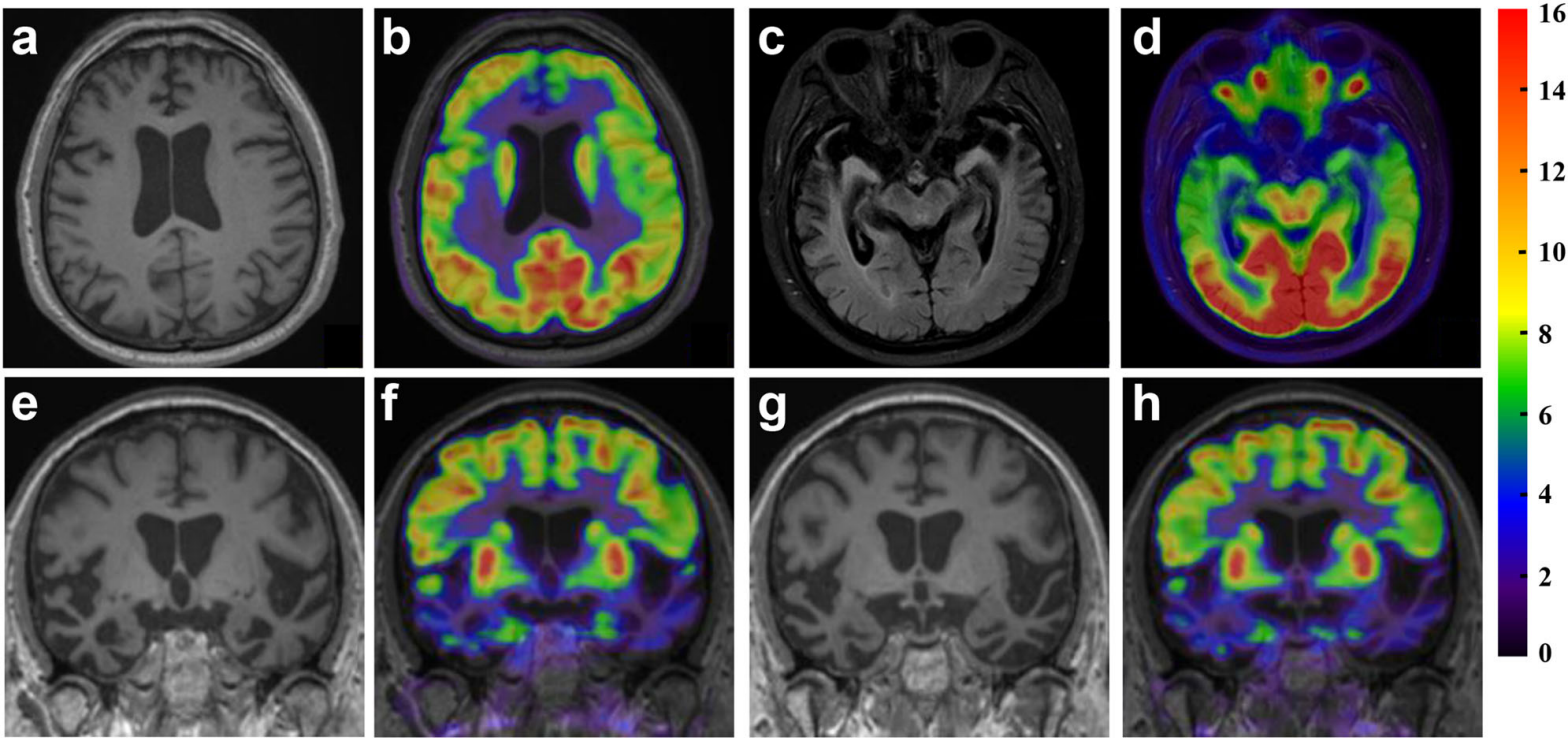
^18^F‐FDG PET/MR images of patient 3 Note the obvious hypometabolism, predominantly on the left side, without distinct atrophy in the bilateral frontal lobes (a, b), and severe, “blade‐like” atrophy in the bilateral temporal lobes along with significant hypometabolism, especially in the anterior and lateral parts (c–h)
